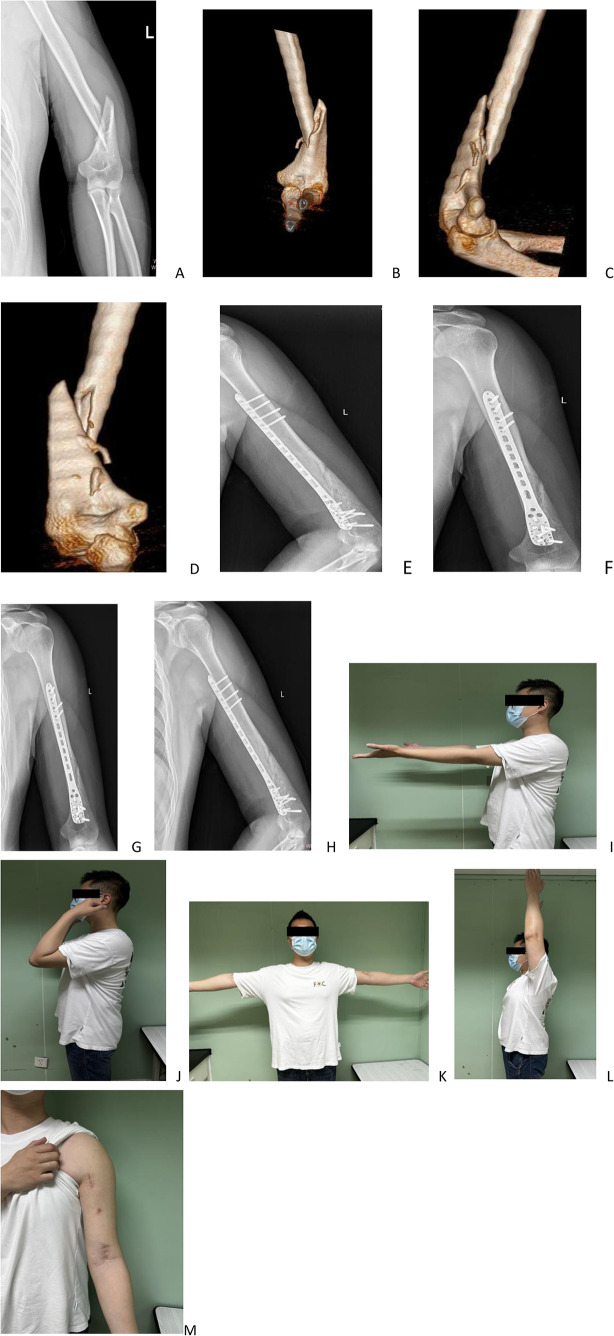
(Patient B): a 31-year-old male, presented with pain in his left upper arm following a fall. Radiographic evaluation, including X-ray and CT imaging, confirmed a spiral fracture of the humerus (A-D). The fracture was treated using an inverted PHILOS plate fixation via an anterior minimally invasive surgical approach (E-F). A follow-up X-ray taken two months postoperatively demonstrated satisfactory fracture alignment and healing progress (G-H). At follow-up, the patient’s left elbow exhibited a functional range of motion from 0° to 135°. The patient achieved a Mayo Elbow Performance Score (MEPS) of 95 and a Constant-Murley shoulder score of 98, reflecting a satisfactory functional recovery (I-L). The total length of the surgical incisions was approximately 13 cm, and the incisions healed without complications (M)
